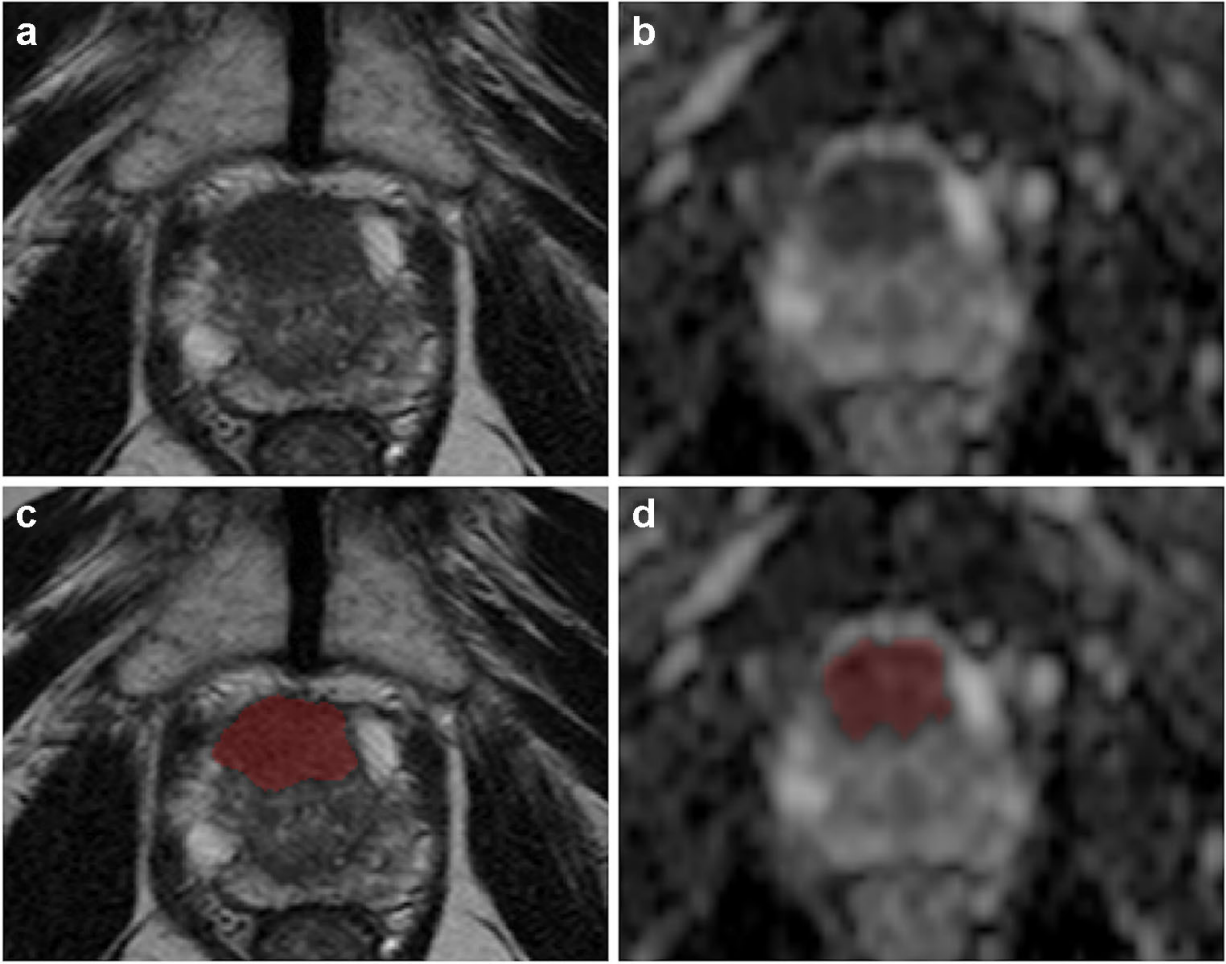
Prostate MR images (axial T2-weighted on the left and ADC map on the right) from a 76-year-old patient with a PI-RADS 5 transition zone lesion involving the anterior fibromuscular stroma (Gleason score 4+3 and signs of extraprostatic extension of disease at prostatectomy). The slices in which the lesion was more conspicuous are shown respectively before (a and b) and after (c and d) after manual segmentation
